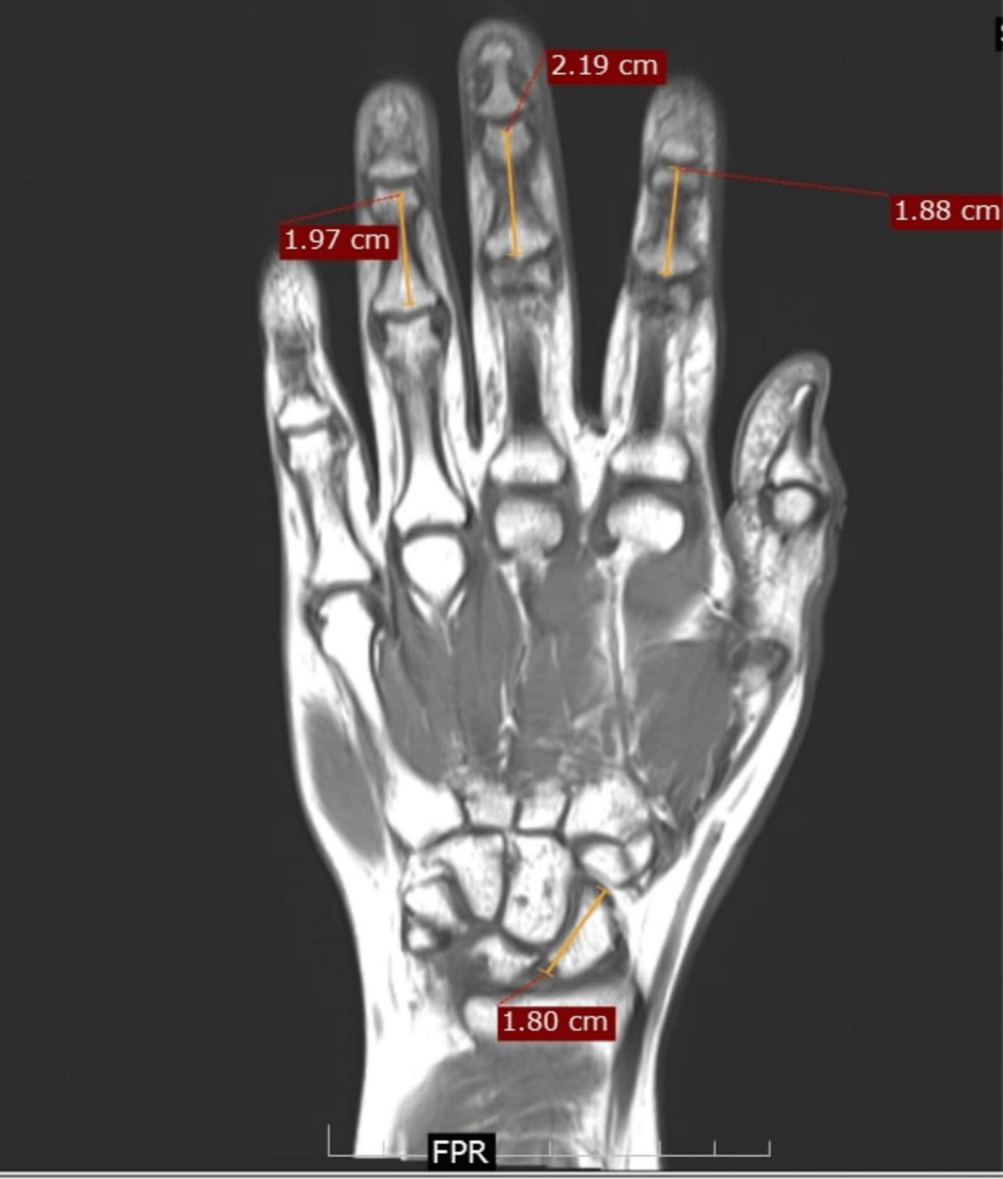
Coronal T1-weighted MRI section of the hand and the wrist showing the method to measure the length of the middle phalanges and the scaphoid.
